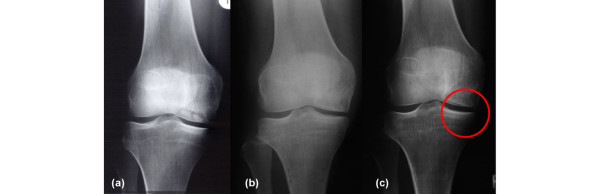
Progression of osteoarthritis in X-ray examinations. Progression of osteoarthritis in Patient 6: (a) 5 months before surgery, (b) 12 months postoperatively and (c) 72 months postoperatively. The circle marks the medial compartment without osteoarthritic changes.
